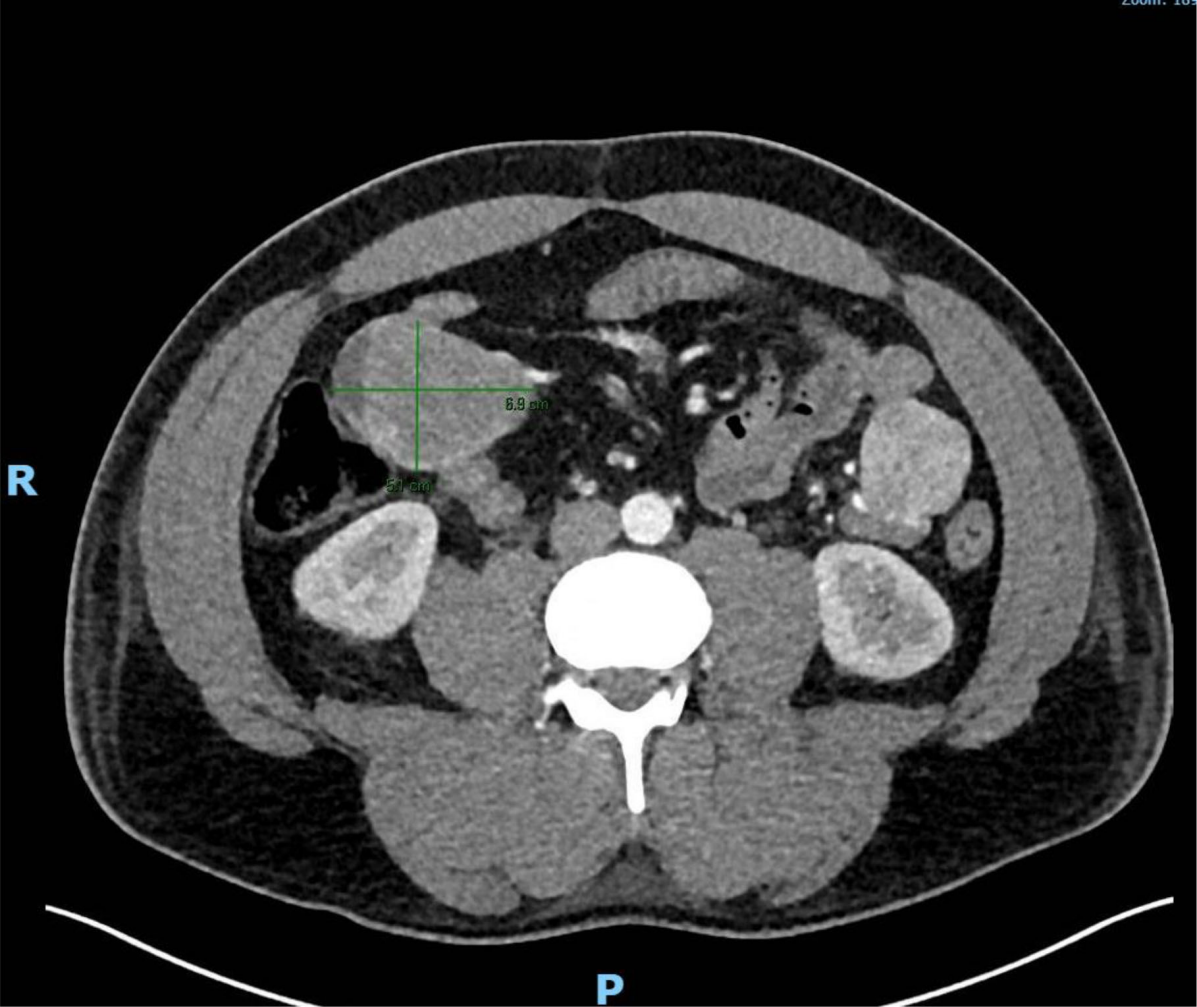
Largest hypervascular lesion sized 69 × 51 mm noted on CT angiogram of abdominal aorta (marked on image) in duodenum. Patient was noted to have 11 other hypervascular lesions in his duodenum.
